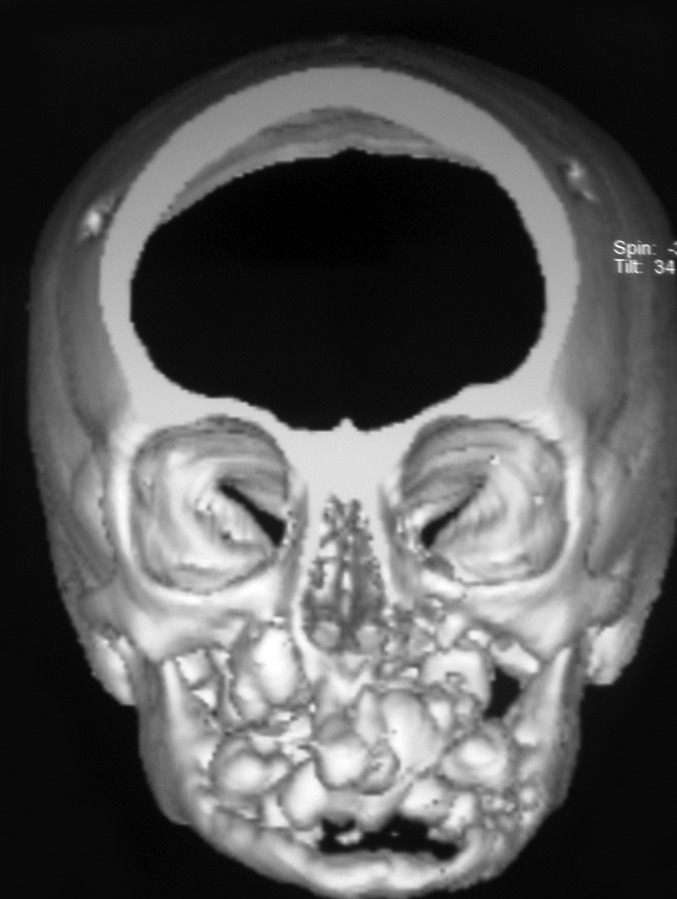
3D constructive view of CT-head
